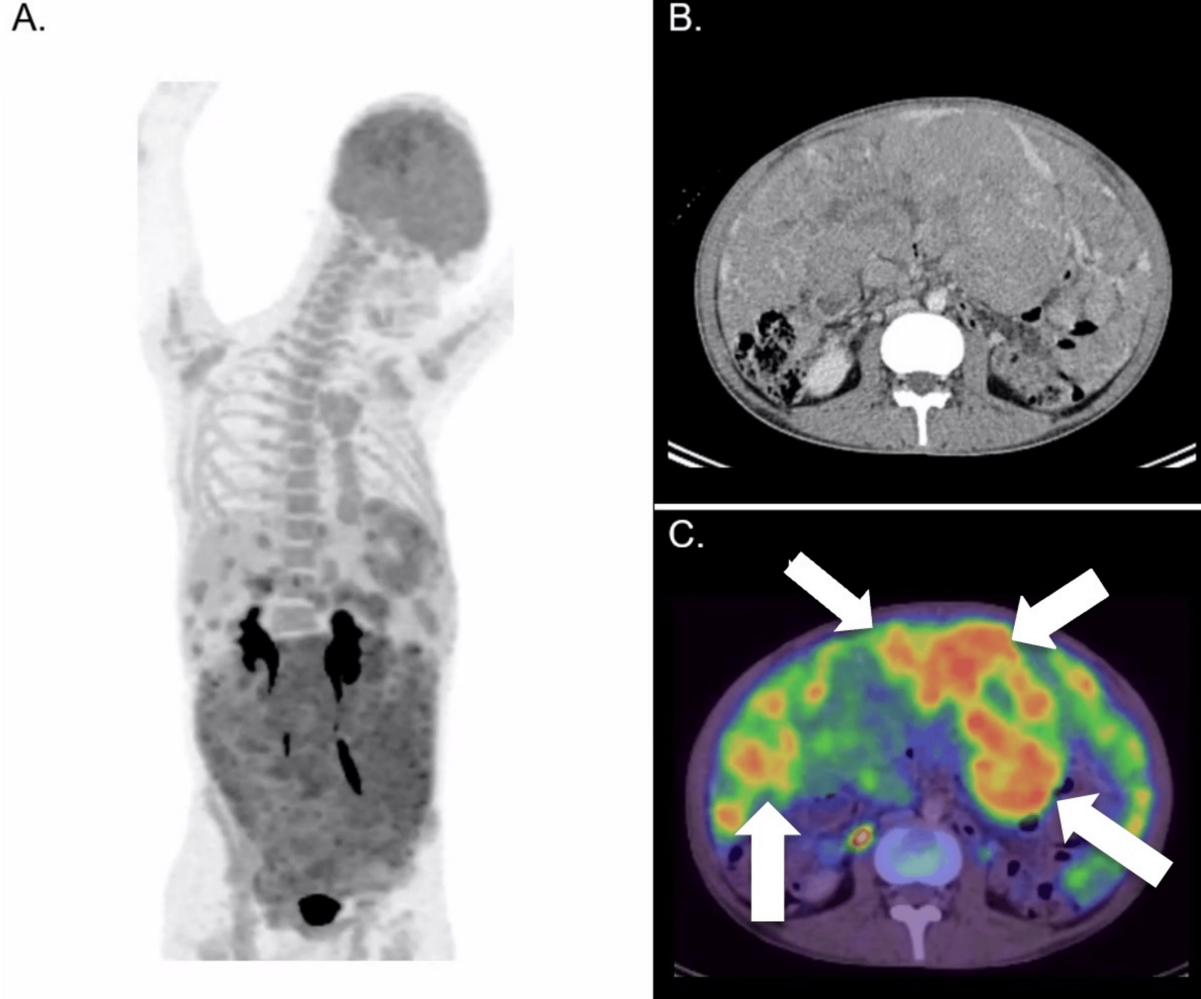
Follow-up 18F-FDG PET/CT scan (two months from the initial PET/CT scan) After two months of systemic chemotherapy, follow-up imaging was performed to assess the treatment response. MIP (3A), axial contrasted CT (3B), and fused PET/CT images (3C) were acquired. The slight reduction in the size of the peritoneal and hepatic masses was challenging to appreciate on follow-up CT images. However, the fused PET/CT images more easily demonstrated a decrease in metabolic volume (arrows), indicating a partial metabolic response to the therapy. 18F-FDG PET/CT: 18F-fluorodeoxyglucose positron emission tomography/computed tomography; MIP: maximum intensity projection
